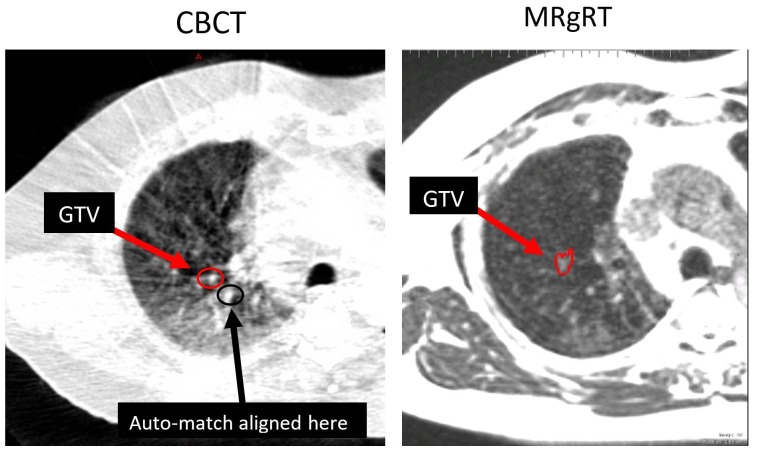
CBCT (left) and MRgRT scan (right). CBCT auto-match would have led to misalignment of GTV due to excessive noise of CBCT. CBCT: cone-beam computed tomography; MRgRT: magnetic resonance-guided radiotherapy; GTV: gross target volume
